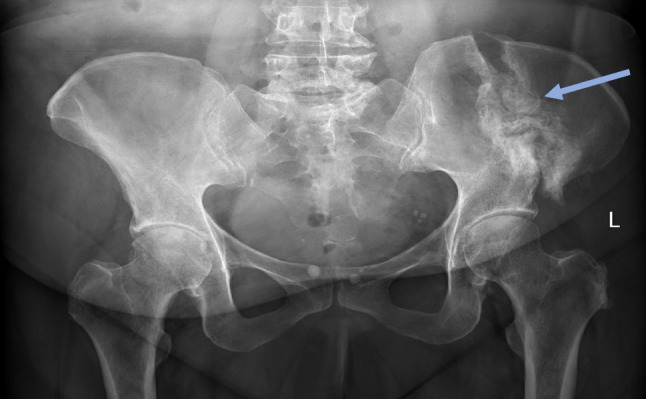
A left iliac wing fracture associated with bisphosphonate use with florid callous formation (blue arrow) after 4 months in a 63-year-old woman. Anteroposterior pelvic radiograph showing florid callous formation at the fracture site (blue arrow)
